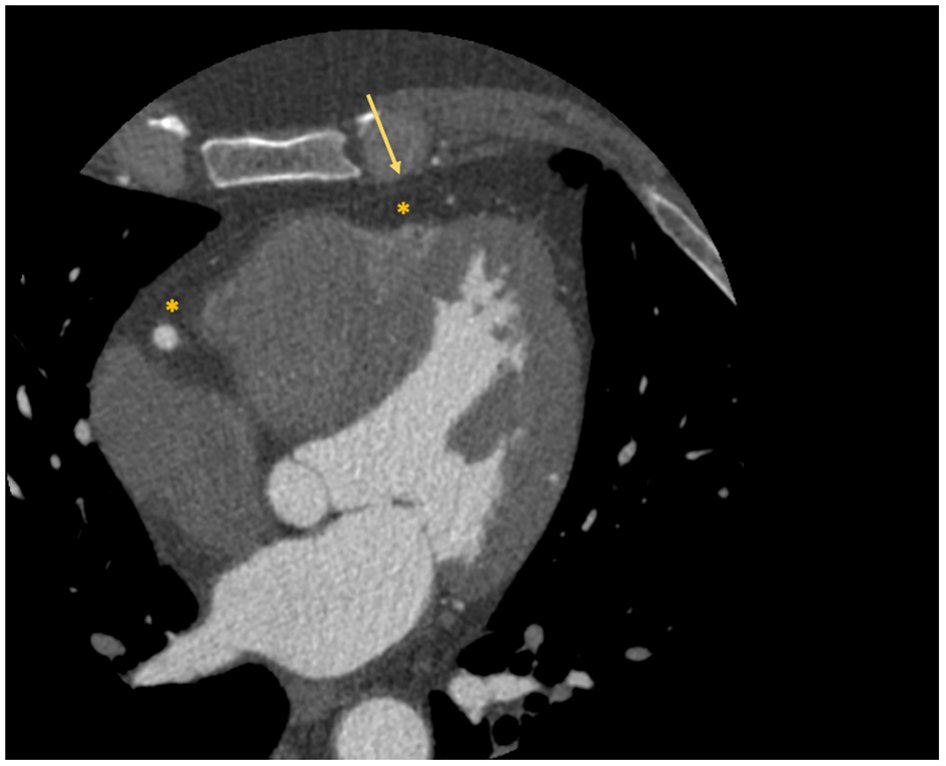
Localization of epicardial adipose tissue at cardiac CT scan. The figure depicts an example of visualization of the epicardial adipose tissue with a cardiac CT scan. The asterisk identifies the hypodense area of adipose tissue; while the arrow identifies the visceral pericardium.
